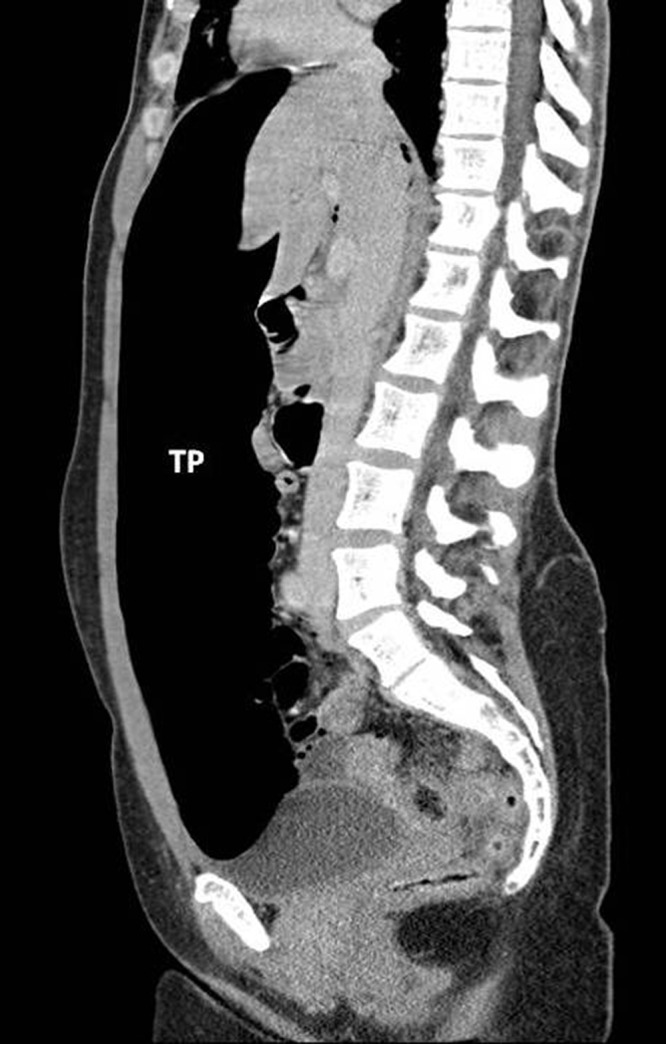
Computed tomography with oral contrast showing pneumoperitoneum with compression of intra-abdominal viscera
